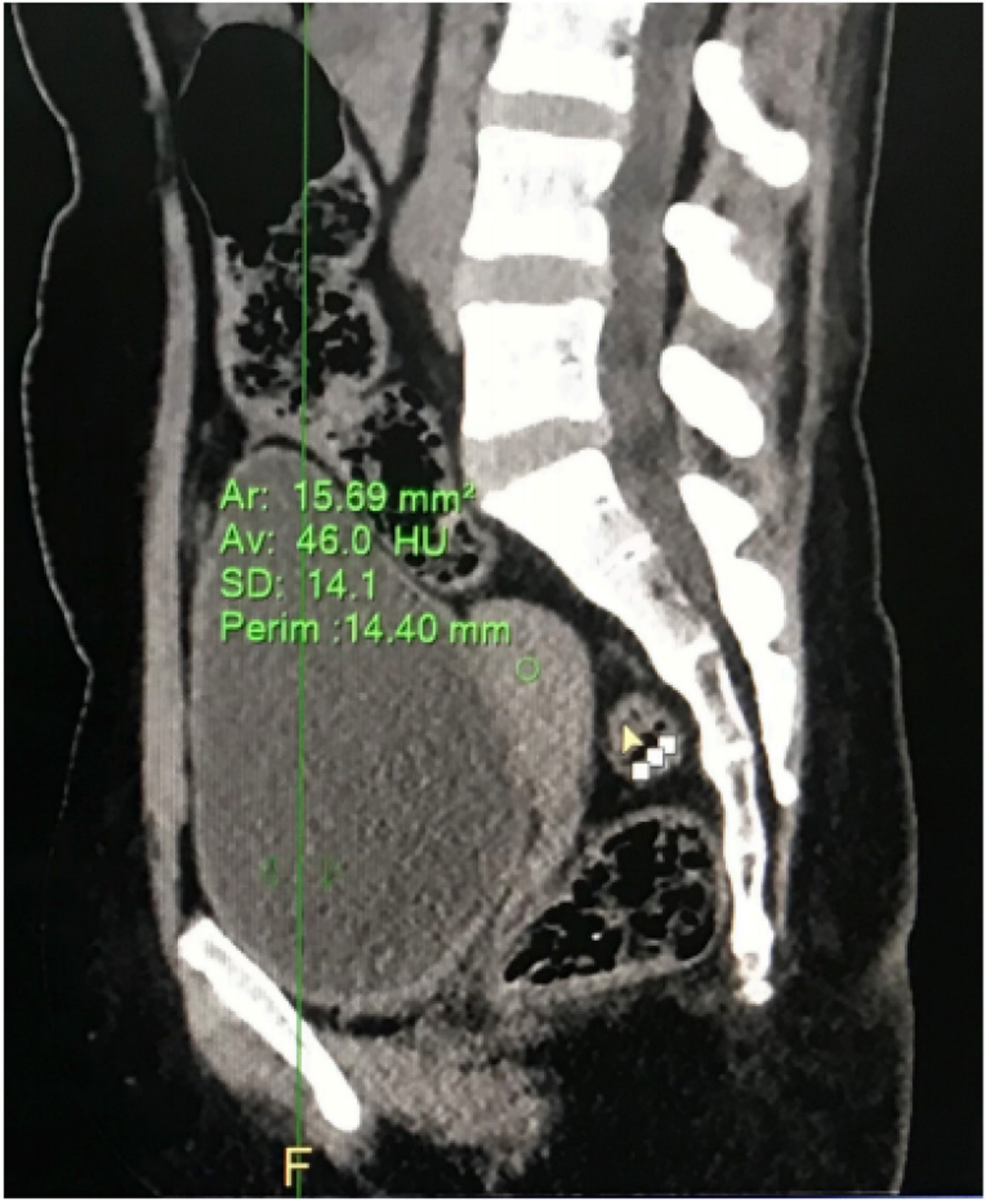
Sagittal CT image showing small sized uterus with diffusely thinned out endometrium and collection within endometrial cavity (HU+46)
